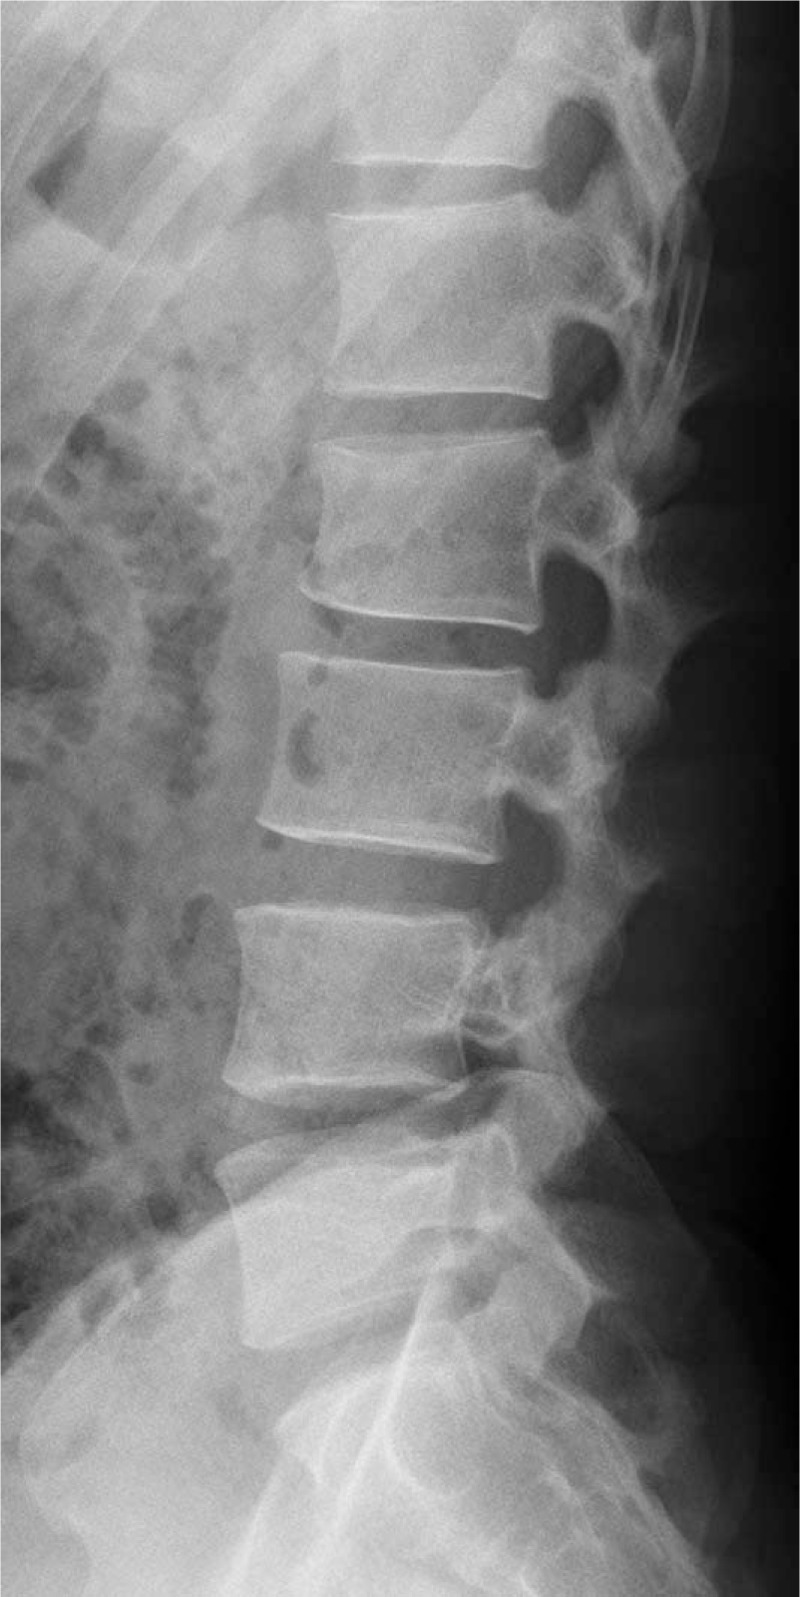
Initial X-ray scan showing a suspected anterior compression fracture of the L2 body.
